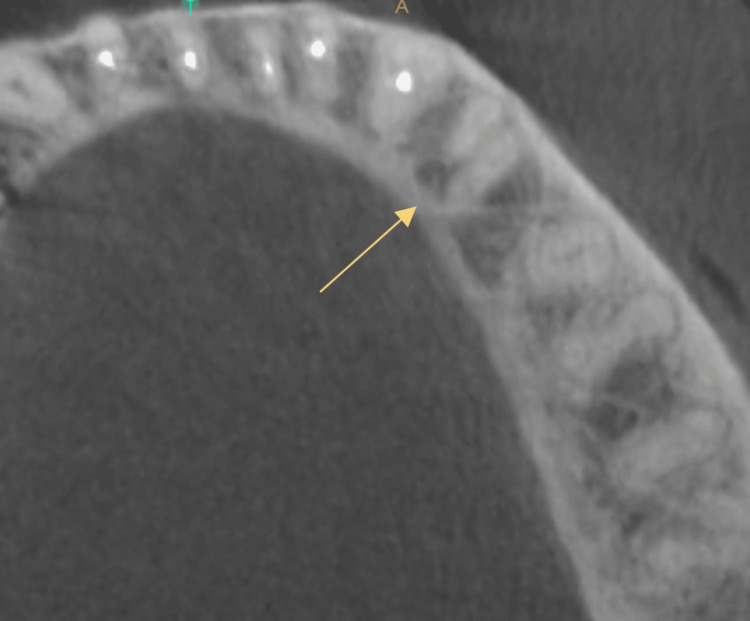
Pre-operative CBCT image of mandibular left first premolar of case 2
